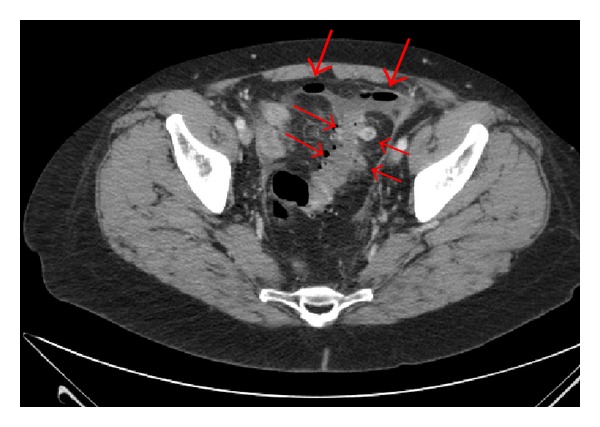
CT scan of perforated diverticulitis with diverticula (thin arrows) and free abdominal air (thick arrows).
